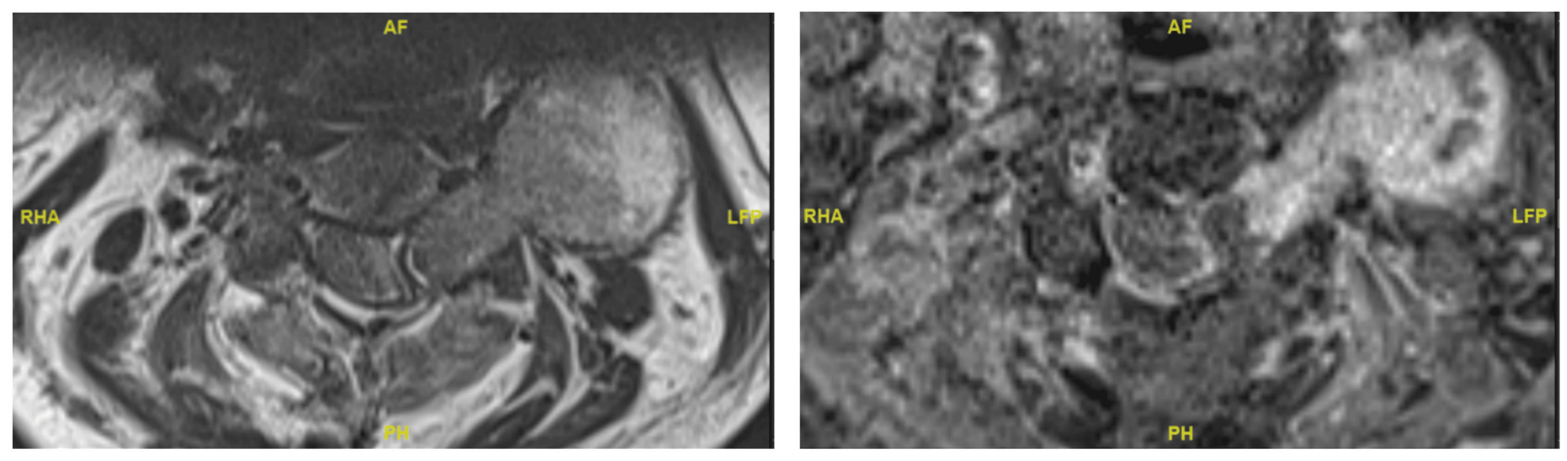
Post-operative axial MRI images of intradural extramedullary lesion at C3-4 levels showing interval increase in space available for cord and notable absence of metal artefacts.
